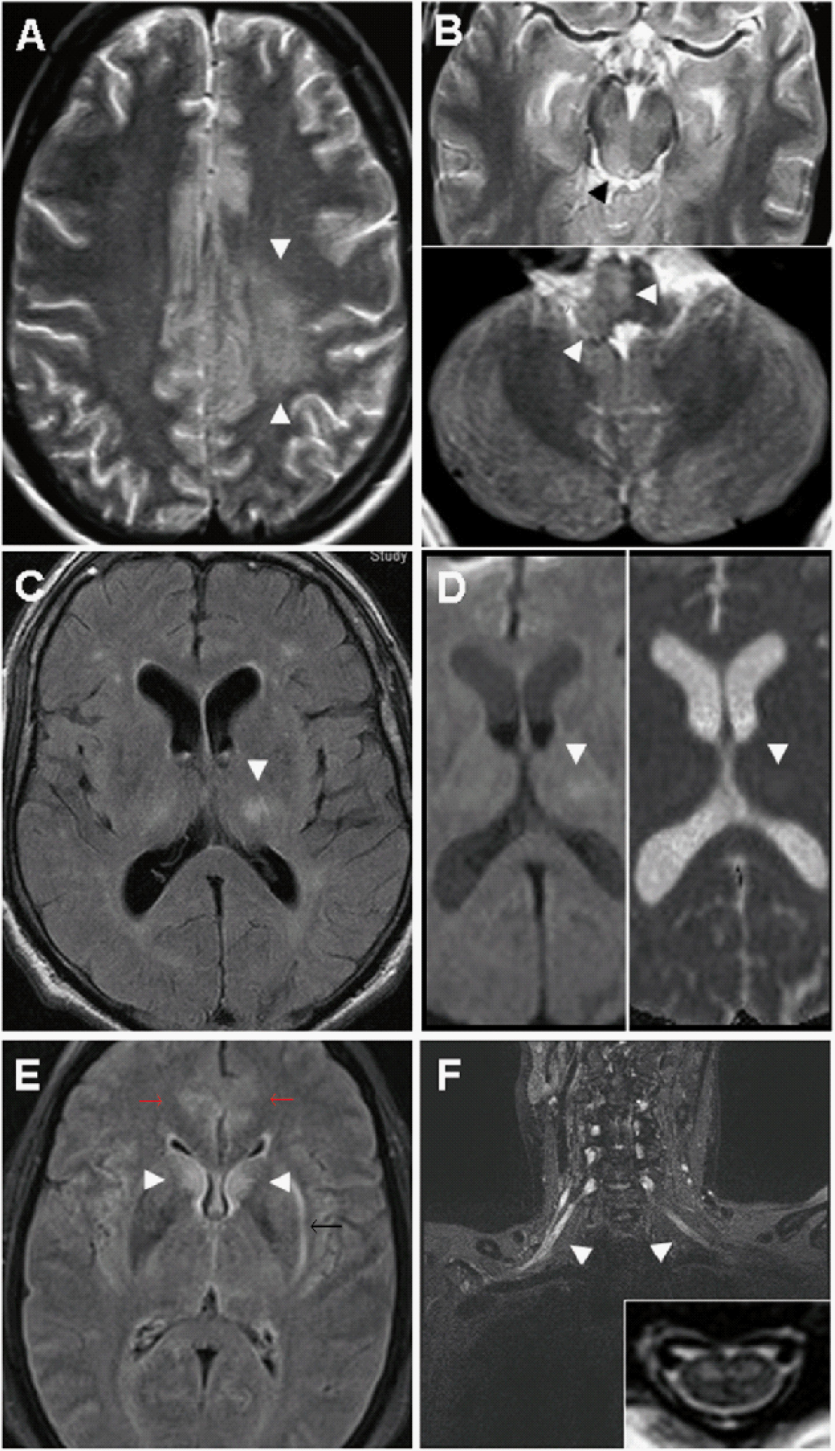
Neuroimaging findings in severe TBE. (A and B), A 23-year-old male patient with severe ME and extensive panencephalitis but complete recovery after 12 months. MRI at admission showed T2-weighted axial lesions of the left semioval centre (A, arrowhead), right inferior colliculum tecti (B, upper panel) and right medulla oblongata (B, lower panel) Not shown: additional T2-weighted lesions in the thalamus and cerebellar peduncles. (C and D), A 62-year-old male MER patient initially misdiagnosed as lacunar stroke with consciousness disturbance, complete hemiplegia, dysphagia and lethal course due to aspiration pneumonia: axial fluid-attenuated inversion recovery (FLAIR) sequence depicts a rounded hyperintense lesion in the left thalamus (arrowhead) (C) that shows pathological water diffusion on diffusion-weighted imaging (DWI) (D, upper panel). Corresponding ADC map identifies the lesion as vasogenic oedema with enhanced water diffusion (D, lower panel), incompatible with acute or subacute stroke. (E and F), A 49-year-old female MER patient with “man in the barrel syndrome” (unable to move her upper limbs) as long-term outcome. MRI at admission showed extended encephalitis, cervical myelitis and brachial radiculitis: axial FLAIR image depicts bilateral hyperintense lesions of caudate nucleus (arrowheads), cingulate cortex (red arrows), and left external capsule (black arrow) (E). A T1-weighted, fat-saturated coronal image of the brachial plexus shows bilateral contrast enhancement of the displayed nerve roots and fascicles, proving radiculitis (F). Inset: A T2-weighted axial image of the cervical myelon shows bilateral, symmetrical, hyperintense lesions strictly confined to the anterior horn.
